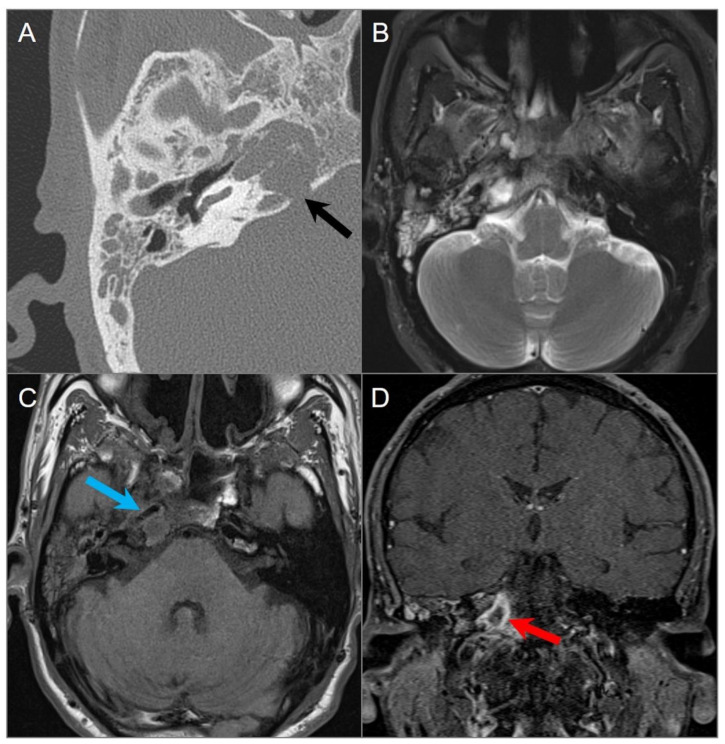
Petrous apicitis. (A) Temporal bone CT image shows opacification of the right mastoid air cells and external auditory canal with thickening of the tympanic membrane consistent with external otitis and mastoiditis. There is opacification of the right petrous apex with dehiscence of its posteromedial margin (black arrow). (B) Axial T2W and (C) noncontrast T1W images demonstrate fluid in the mastoid air cells, middle ear cavity, and petrous apex with associated thickening of the carotid artery wall, resulting in mild luminal narrowing (blue arrow). (D) Coronal postcontrast fat-suppressed T1W image reveals a peripherally enhancing collection within the right petrous apex consistent with abscess (red arrow).
